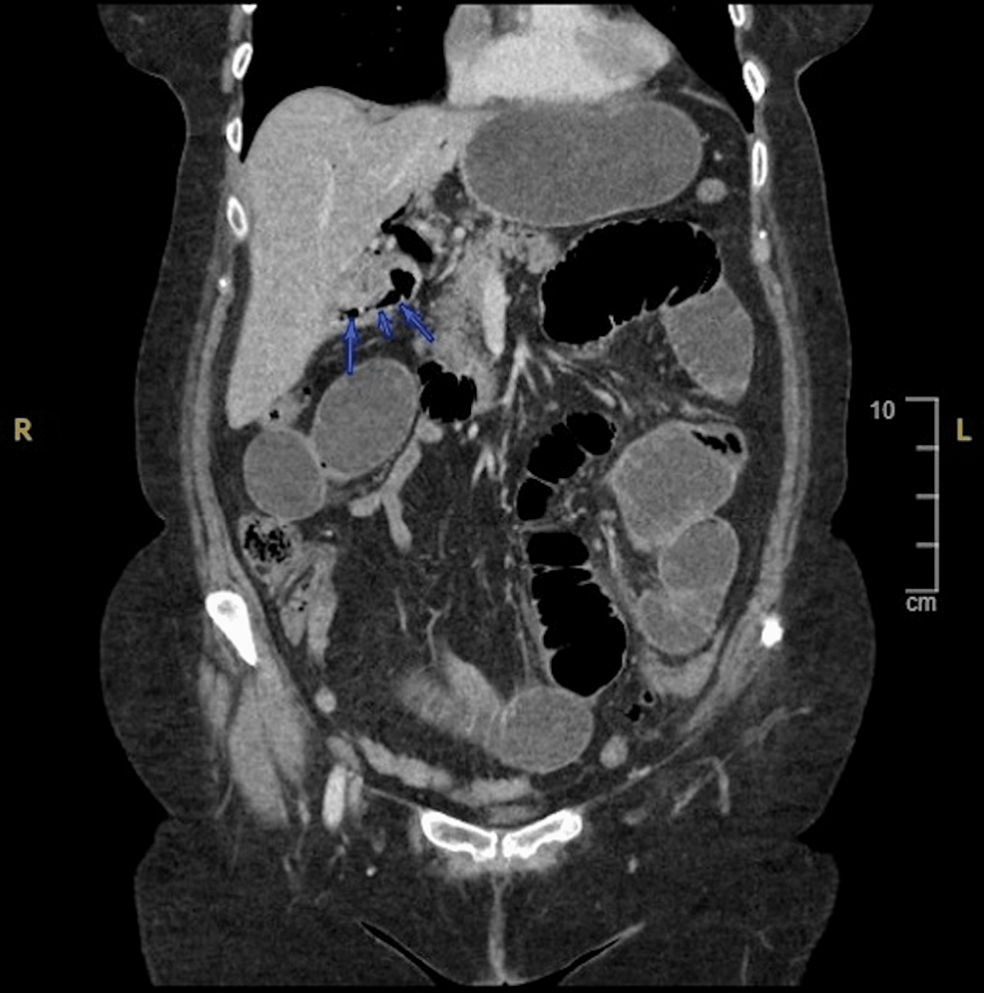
Computed tomography scan coronal view of abdomen showing gas in biliary tree and gallbladder.
